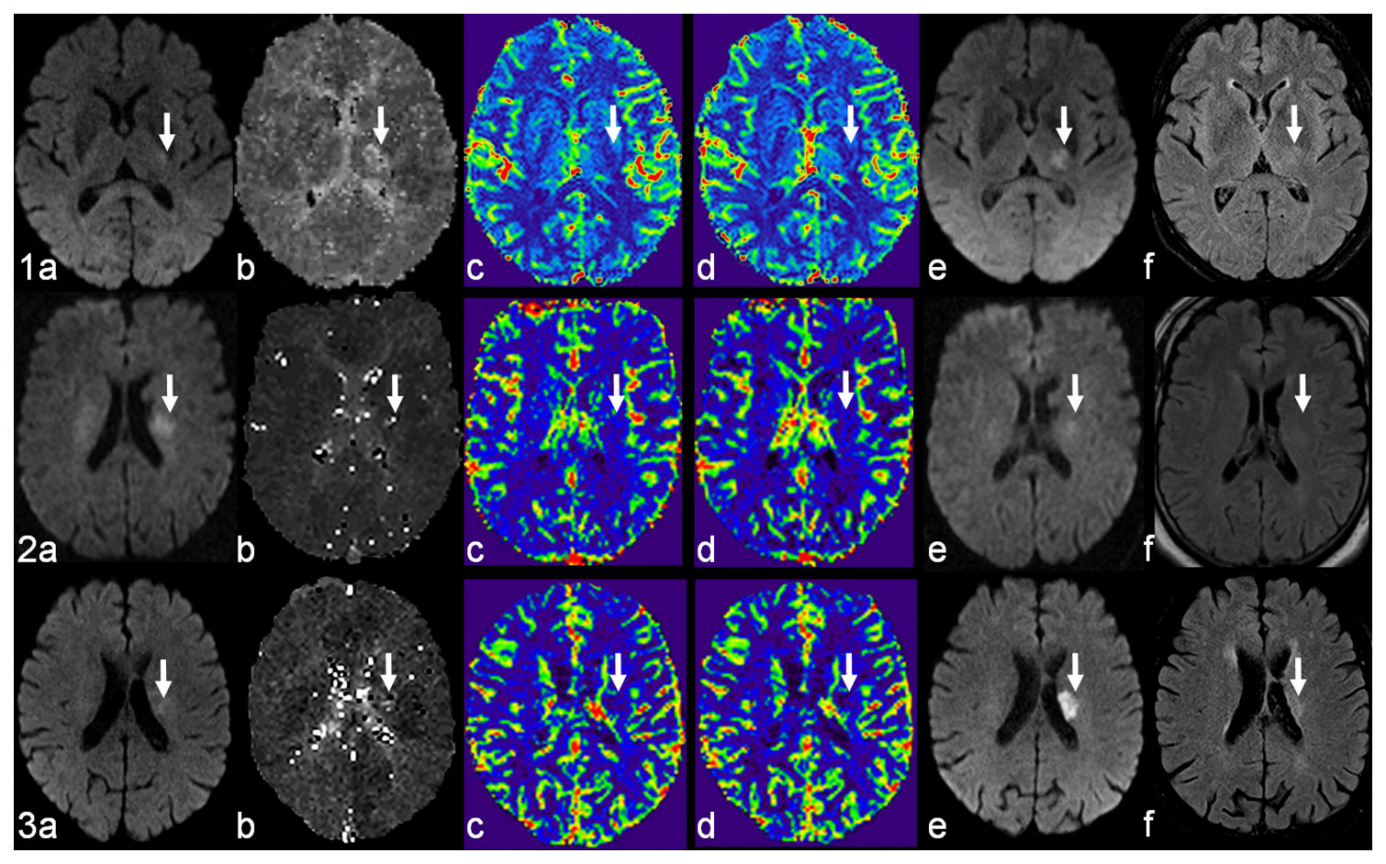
Three examples of perfusion patterns in acute lacunar infarction. Mismatch (1), inverse mismatch (2), and match (3) between diffusion-weighted and perfusion-weighted images (DWI, PWI). DWI (a) shows the initial ischemic lesion (white arrow). PWI derived maps demonstrate the perfusion deficit: time to peak (b), cerebral blood flow (c), and cerebral blood volume (d). Follow-up DWI (e) and FLAIR (f) show the ischemic lesion (white arrow). Note that case 2 is also an example of lesion reversal after intravenous thrombolysis.
